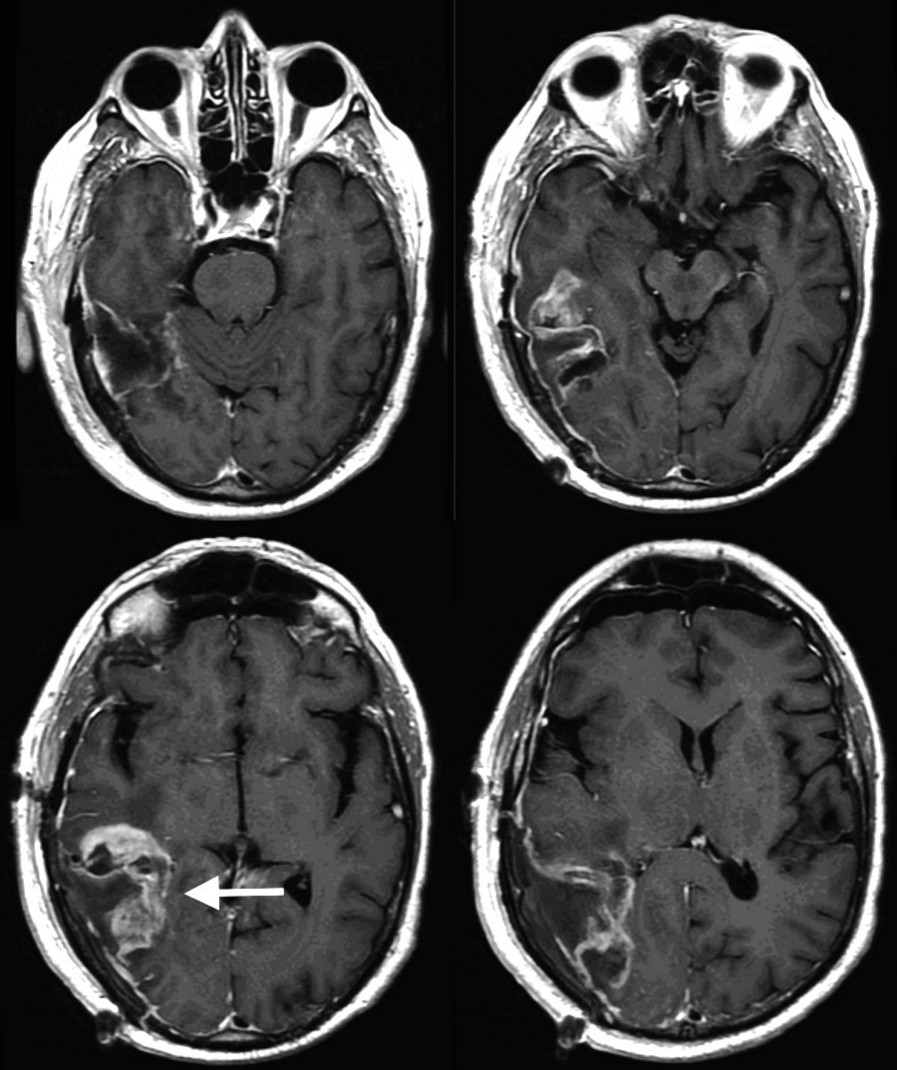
Post-operative axial T1 post-contrast MRI Post-operative surgical cavity with residual tumor anterior and medial to the resection margins (arrow) MRI: magnetic resonance imaging
